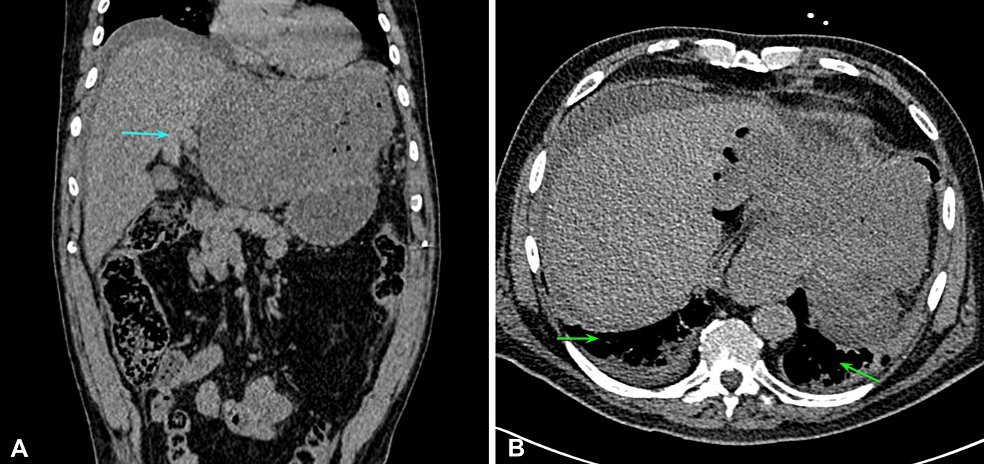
Non-contrasted abdominal CT scan. Esophagogastric junction with prominence of gastric folds through the hiatus: peritoneal free air with perihepatic and perisplenic free fluid is observed (green arrows). A heterogeneous image with air that seems to depend on the lesser curvature of the stomach that loses interface and measures approximately 10x15x12 cm (blue arrow) associated with multiple lymph nodes and peritoneal fatty striation: part of the stomach, body, and antrum are seen displaced downwards. Image A: coronal plane. Image B: axial plane.
